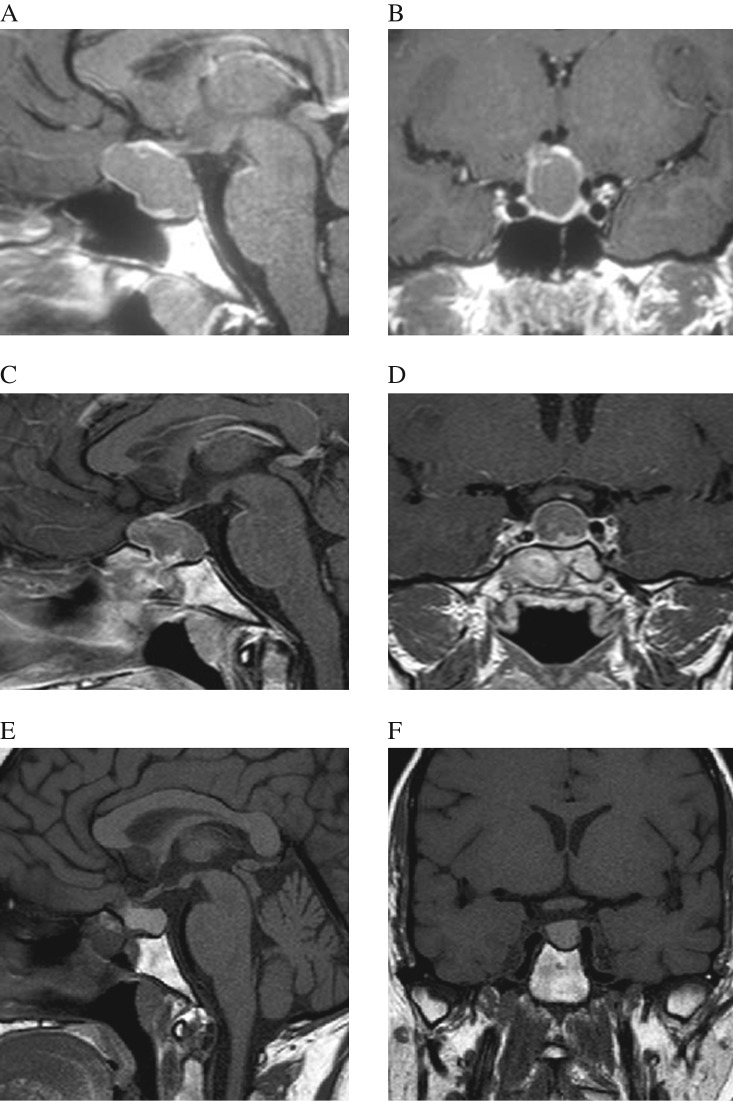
(A and B) Preoperative gadolinium-enhanced T1 weighted MRI: lesion measuring 19 mm vertical length near the optic chiasm, with irregular peripheral enhancement. (C and D) Postoperative gadolinium-enhanced T1 weighted MRI: persistence of heterogeneous asymmetrical mass with infiltration of the sphenoidal sinus near the optic chiasm. (E and F) Nonenhanced MRI: significant reduction in tumor size 4 months after starting meprednisone treatment.
